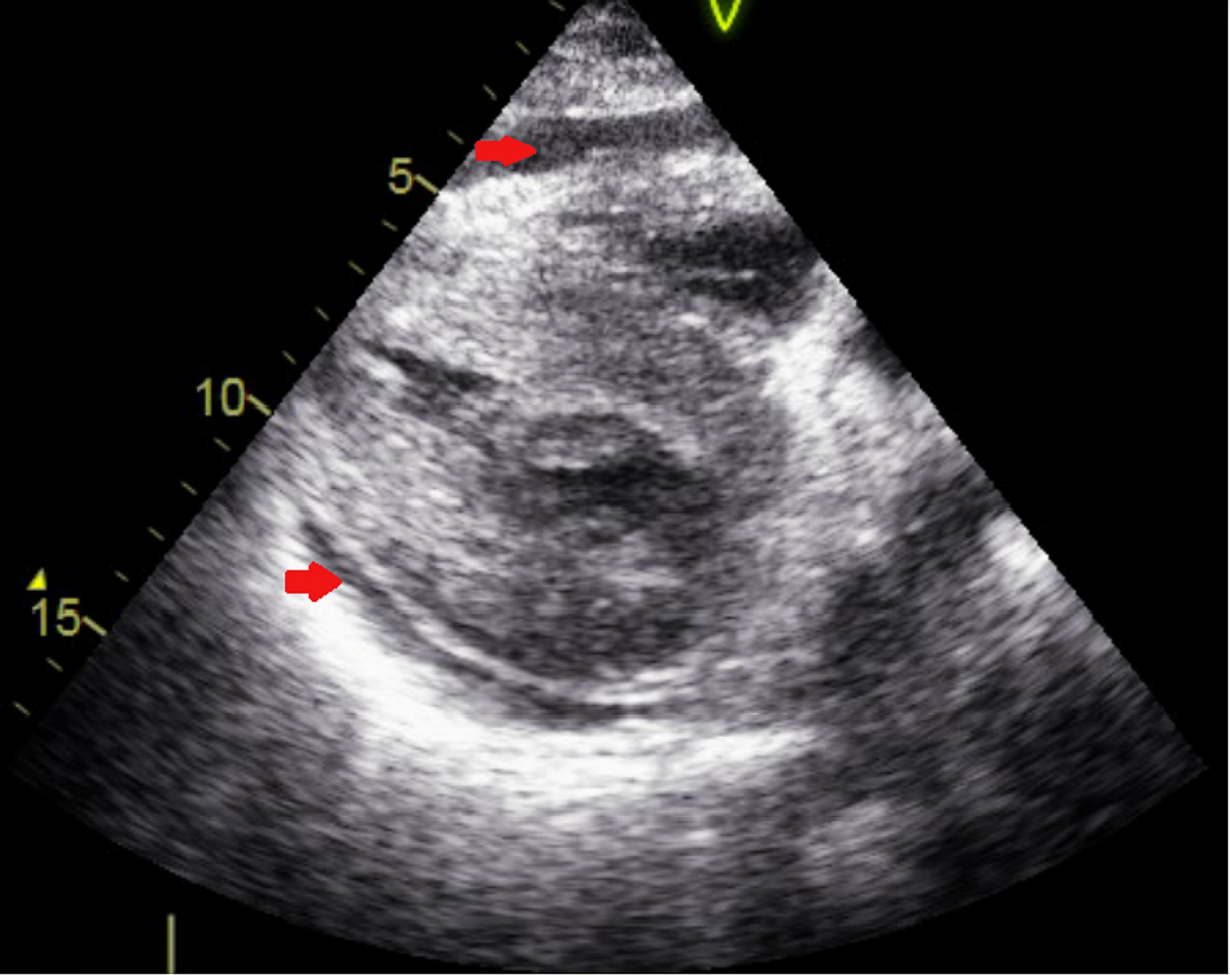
Initial echocardiogram showing pericardial effusion. Red arrows: pericardial effusion
